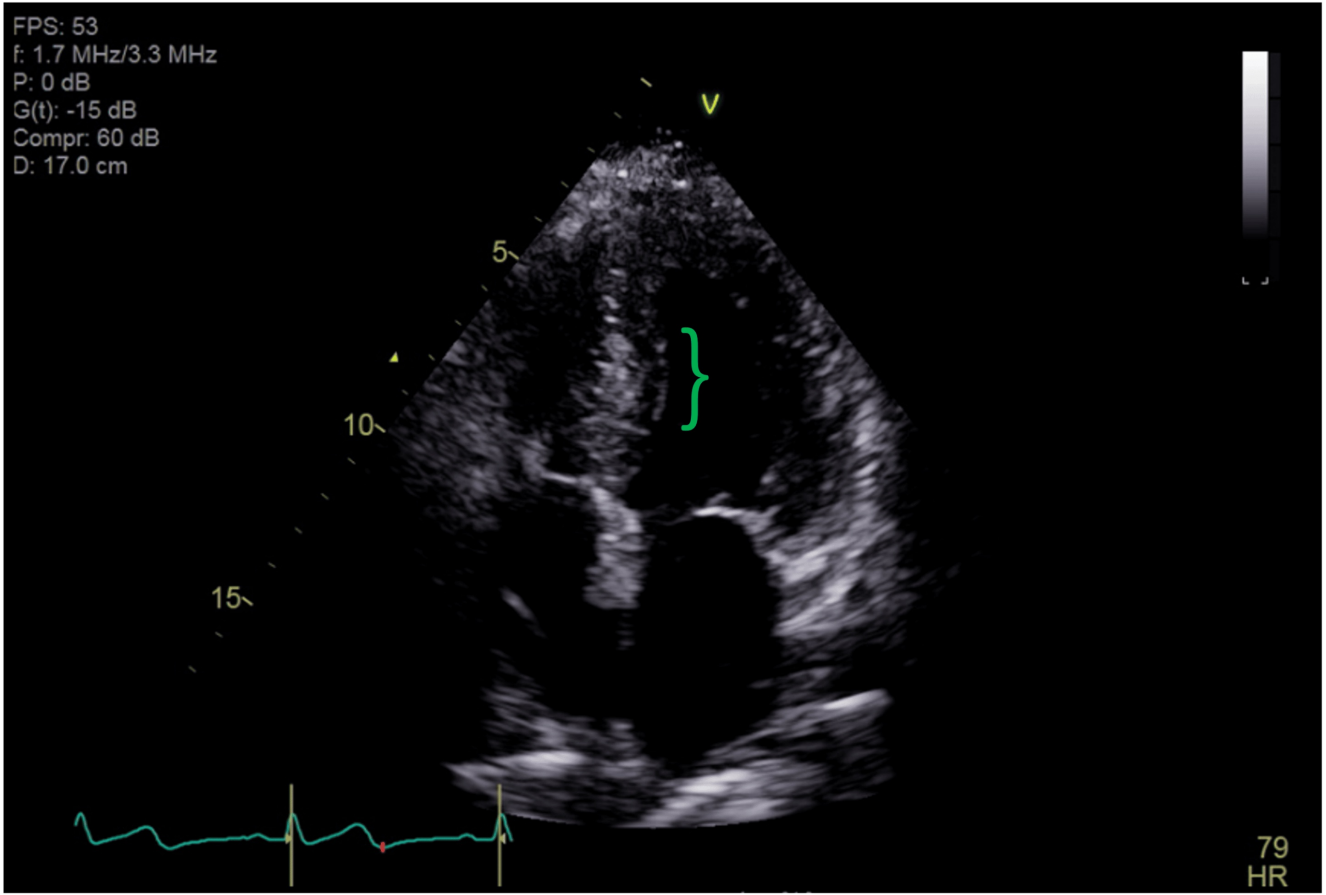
Transthoracic Echocardiogram Transthoracic echocardiogram (TTE) with left ventricular hypertrophy and speckled intraventricular hypertrophy suggestive of cardiac amyloidosis (bracket) [8].
